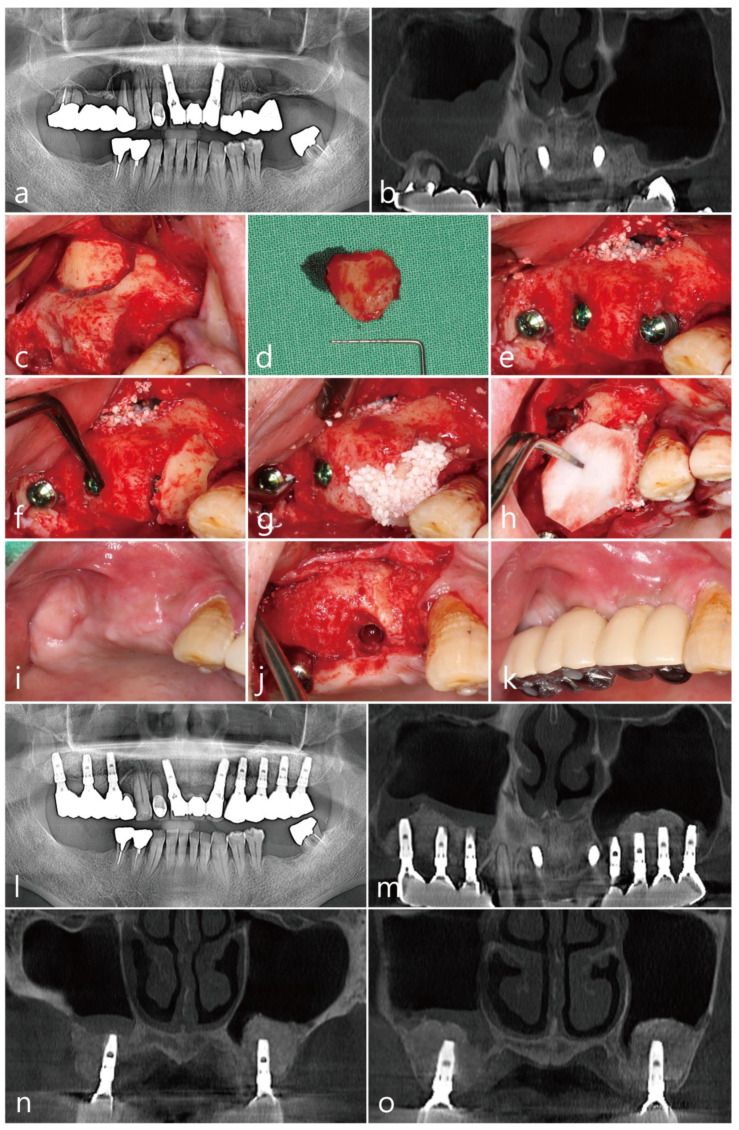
Case 4: (a) preoperative panoramic radiograph; (b) panoramic image of the preoperative CBCT scan; (c) after flap reflection, the lateral bony window was prepared; (d) obtained lateral bony window; (e) implants were placed after the maxillary sinus augmentation; (f) the lateral bony window was cautiously trimmed and placed on the mesial bony defect; (g) additionally, particulate bone graft was used to fill the gap; (h) a resorbable collagen membrane was placed on the grafted site; (i) a clinical view at six months after surgery; (j) the grafted lateral bony window was well integrated with the surrounding native bone; (k) clinical view after prosthesis delivery; (l) panoramic radiograph at six months after prosthesis delivery; (m) panoramic image of the CBCT at six months after prosthesis delivery; (n) coronal image of the CBCT scan showing posterior superior alveolar arteries on both sides; (o) coronal image of the CBCT scan showing reduced mucosal thickening on the right maxillary sinus.
